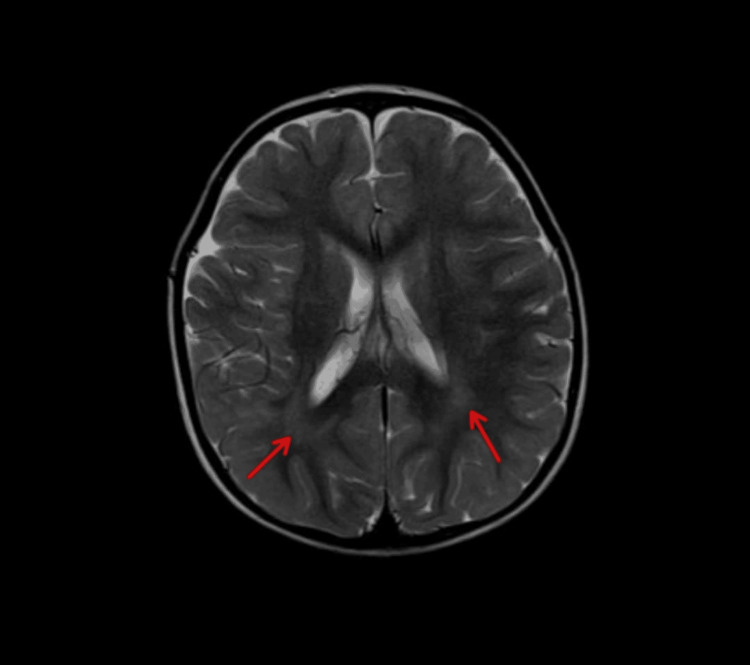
Brain MRI axial view T2 sequence with hypersignal intensities in the occipital head region.
